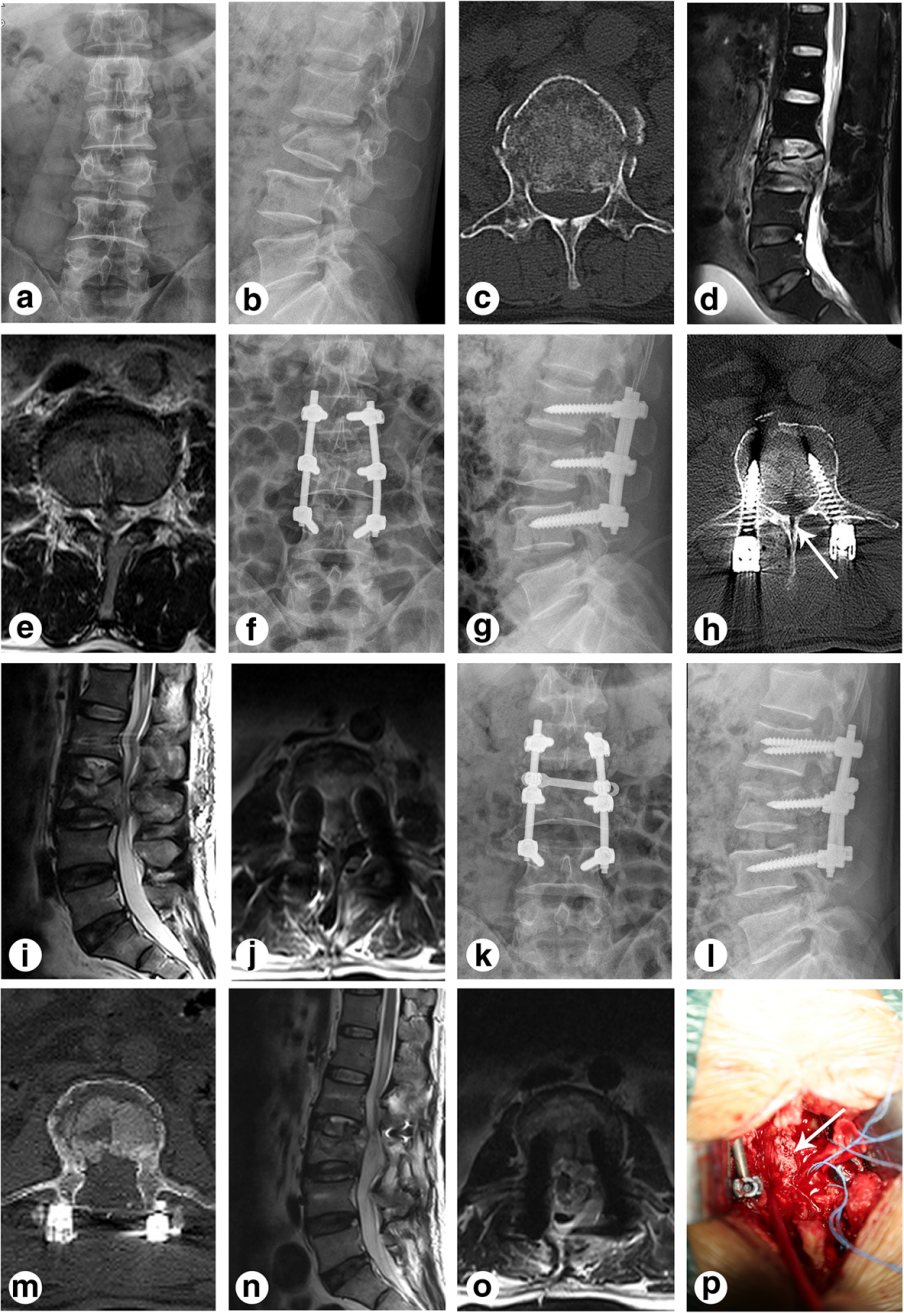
Preoperative, postoperative, and intra-operative graphs of a patient with thoracolumbar burst fractures and greenstick lamina fractures treated with the Wiltse’s paraspinal approach (case 37). a–e X-ray, CT, and MRI before the pedicle screw fixation. f–j X-ray, CT, and MRI after operation. The patient appeared neurological deterioration postoperatively and was offered immediately open book laminectomy surgical treatment. k–o X-ray, CT, and MRI after re-operation. p Dural tears and cauda equina entrapment existed in the patient
